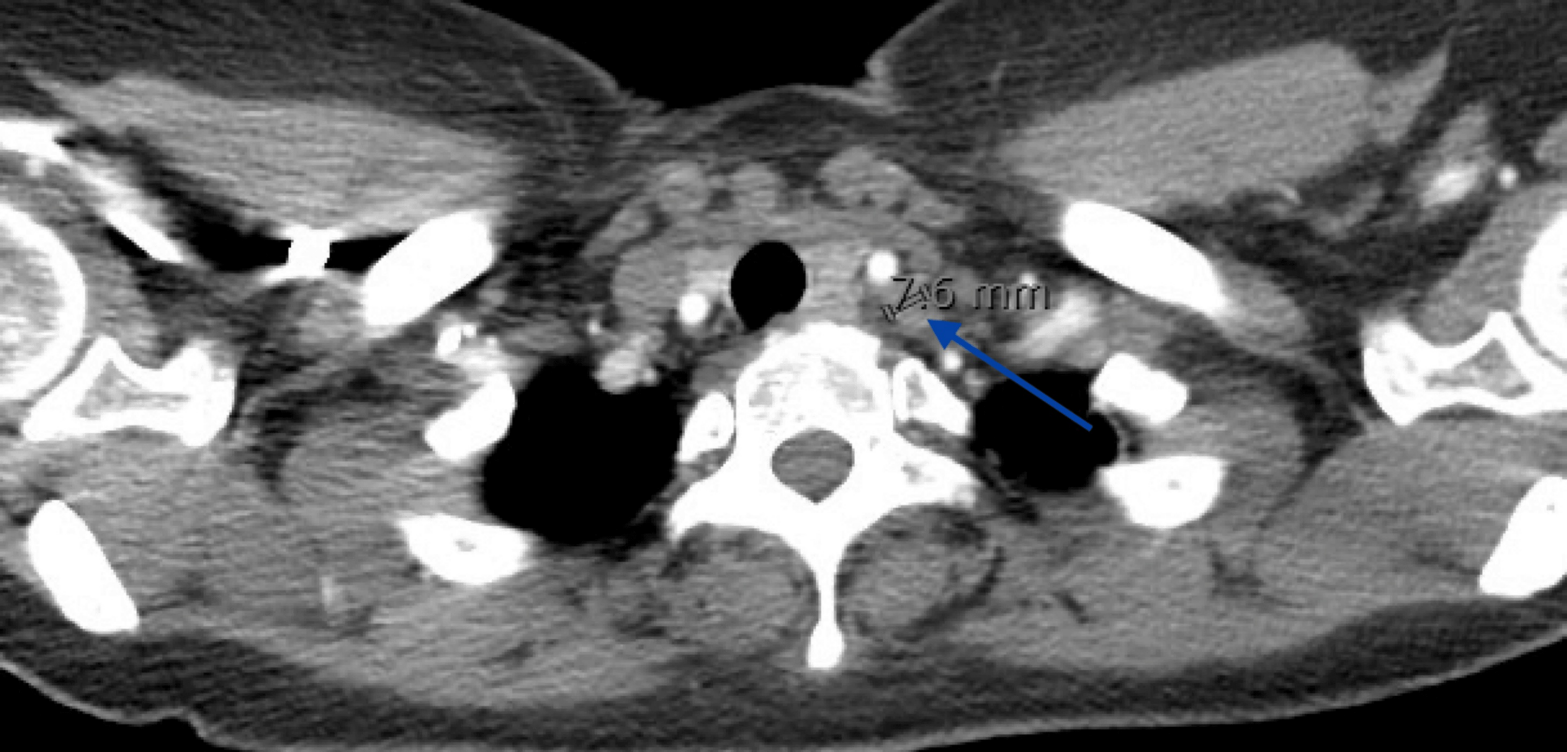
CT angiogram of the chest Blue arrow shows subcentimeter cervical lymph node
